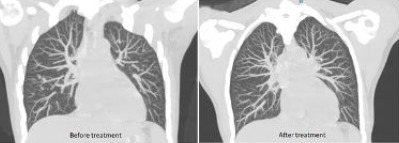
Thoracic high-resolution computed tomography scans before and after antituberculosis therapy.
